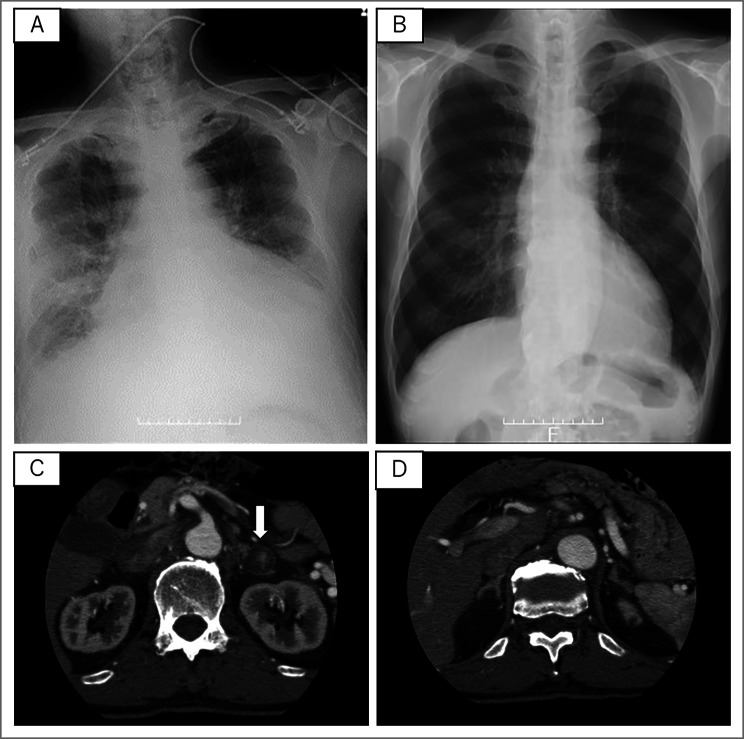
Chest x-ray and adrenal CT images. Preoperative chest x-ray showing pleural effusion and enlarged heart (A). Postoperative chest x-ray showing improvement of pleural effusion and of cardiac enlargement (B). Computed tomography (CT) showing presence of a 25 mm × 23 mm × 22 mm single adrenal nodule on the left gland (C) and absence of adrenal nodule on the right gland (D)
